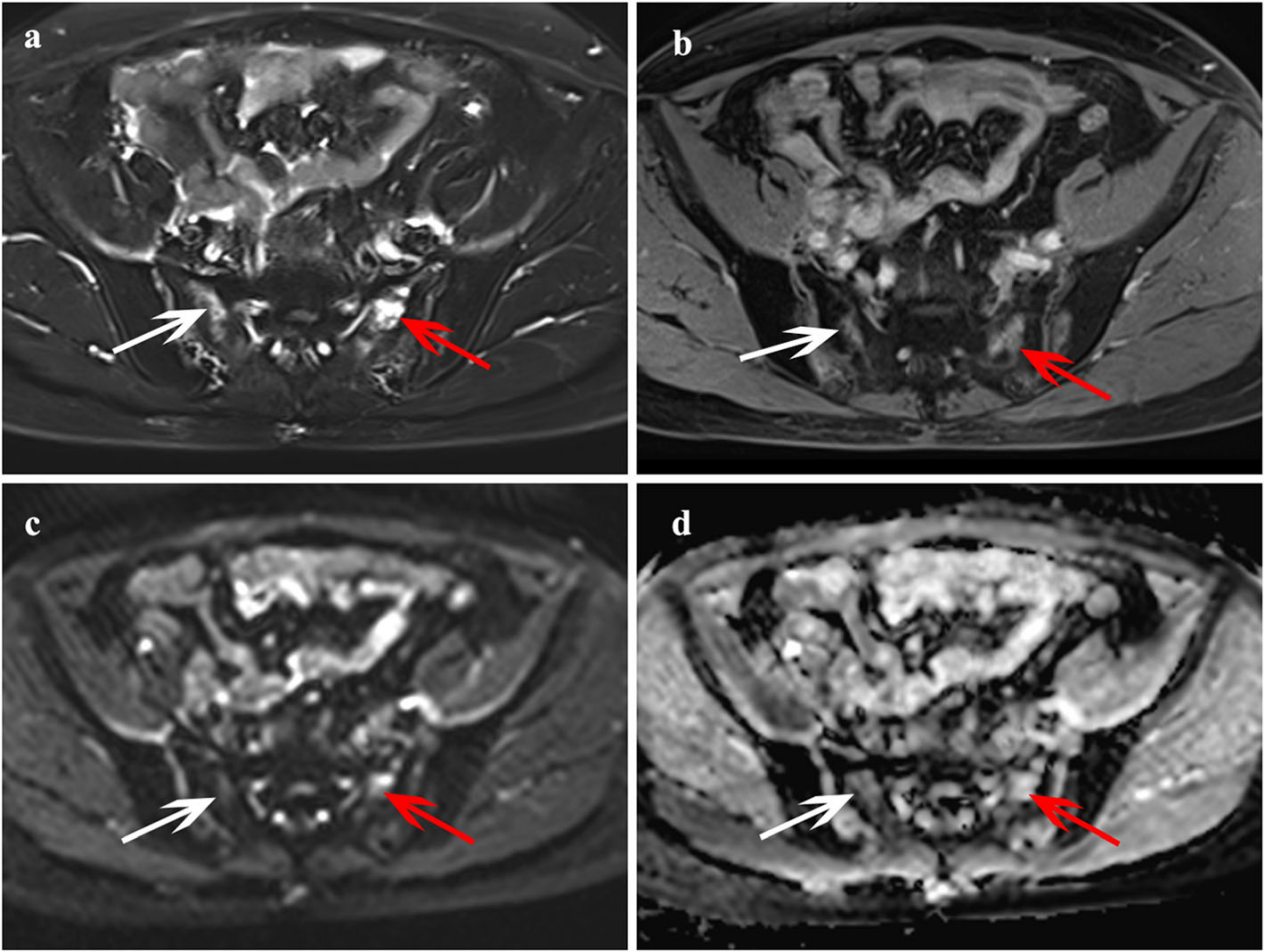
A 52-year-old female with cervical cancer after radiotherapy that was diagnosed with occult IFs (no fracture line detected on all images) in the bilateral sacrum. Axial FS T2-weighted image (a) showed hyperintensity in the bilateral sacrum (red arrow, white arrow, respectively), the lesions revealed contrast enhancement on axial T1-weighted image post-contrast (b). The lesions showed equisignal or slightly high signal intensity on DWI (c), and hyperintensity on ADC map (d)
